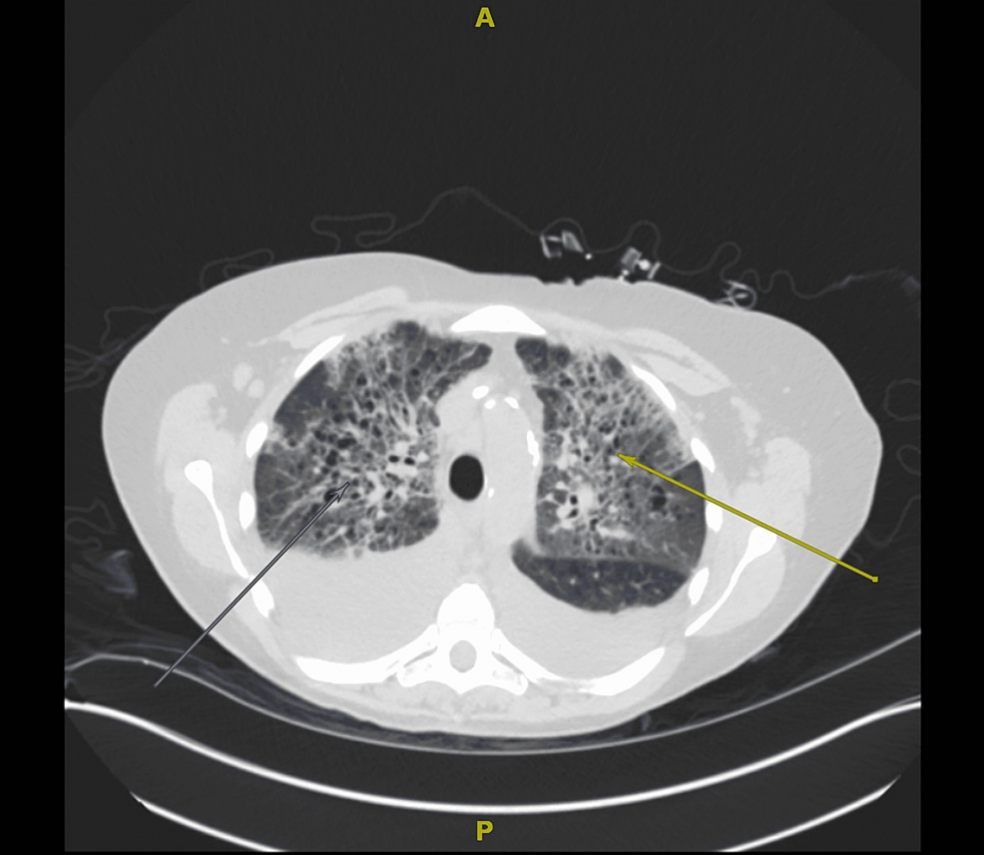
Axial section from a non-contrast CT chest showing large bilateral pleural effusions, interlobular septal thickening, as well as ground-glass and reticular opacities (arrows)
